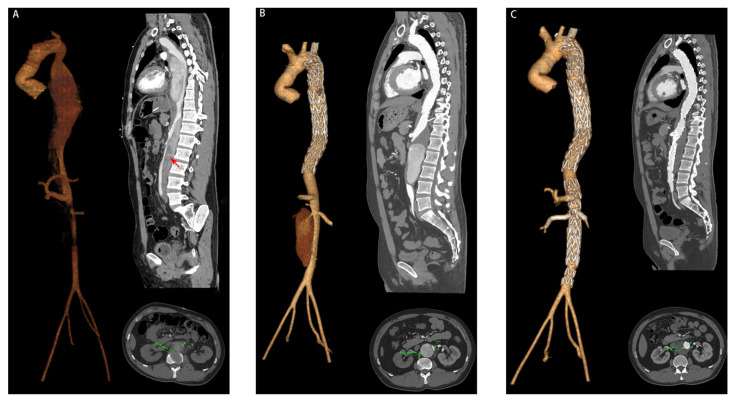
A 43-year-old patient with late aortic expansion at 6 months after TEVAR: (A) aortic CTA at onset of symptoms, where the red arrow indicates partial thrombosis of the FL; (B) aortic CTA at 6 months after TEVAR and a 12.8 mm increase in aortic diameter compared to the preoperative state; (C) aortic CTA at one month after reoperation and complete thrombosis of the FL.
